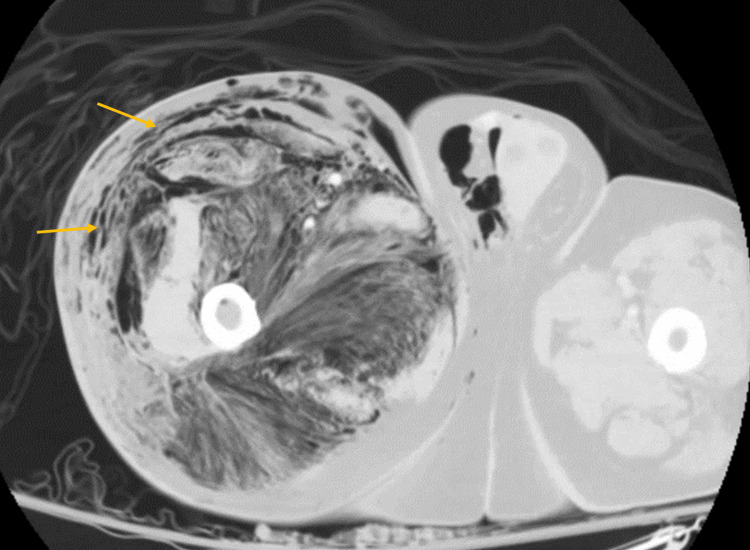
CT image revealing diffuse soft tissue gas present in the right lower extremity The yellow arrows show the diffuse gas present in the right lower extremity CT: computed tomography
